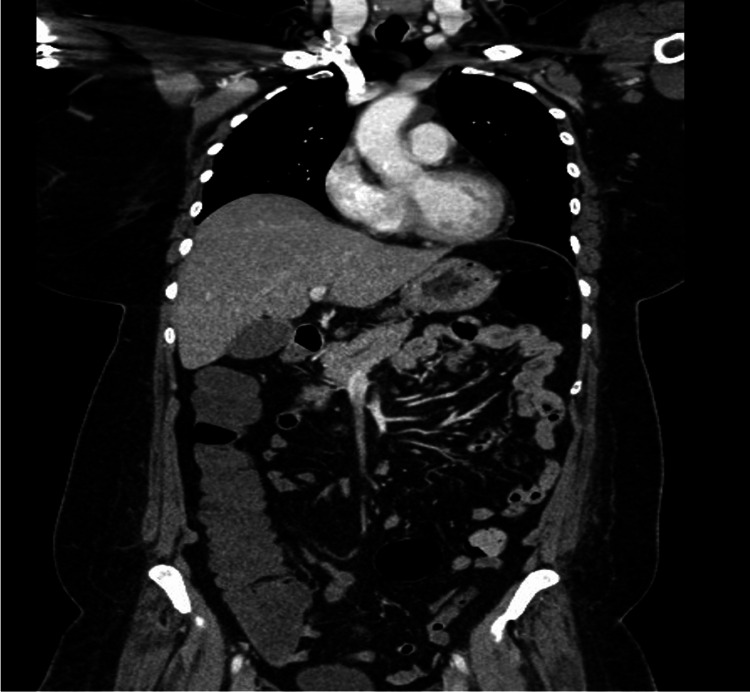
Computed tomography of the chest, abdomen, and pelvis, with contrast in coronal plane No evidence of infection or acute abnormalities was observed in the chest, abdomen, and pelvis
